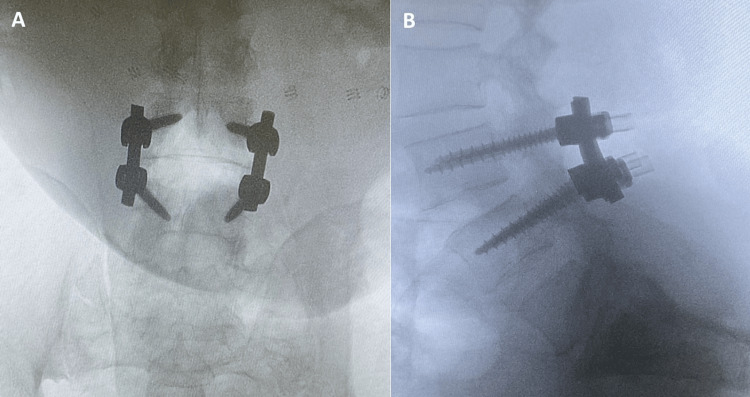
Intraoperative lumbar X-ray Anteroposterior (AP) view (A) and lateral view (B) post posterior decompression with rod and transpedicular screws at L3-L4 level. There were no hardware-related complications. The vertebral body heights and alignment are maintained. Post-surgical changes are noted.
